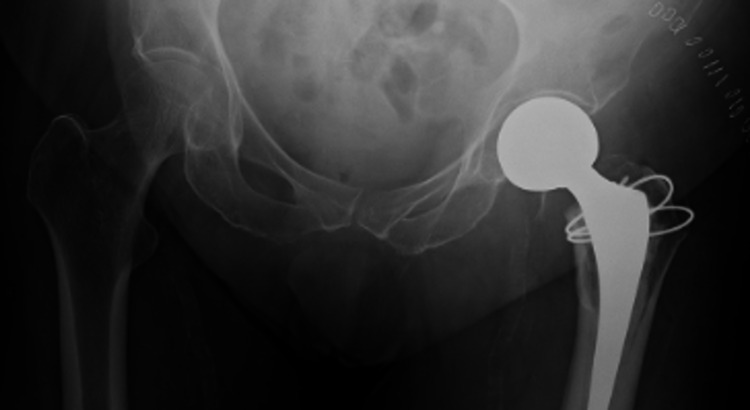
Immediate postoperative radiograph of the patient who subsequently had a prosthetic dislocation This radiograph demonstrates an acceptable prosthesis placement and no concern was raised which would have suggested a future prosthesis failure.
